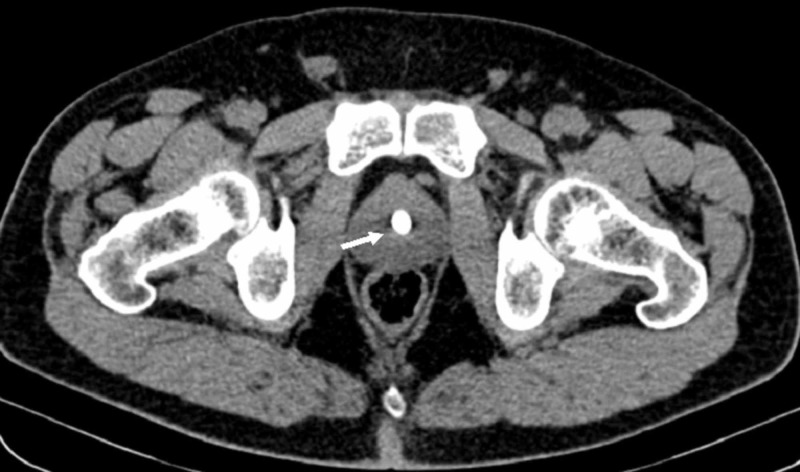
Unenhanced CT scan of the pelvis (axial view) showing the presence of a 9-mm calculi within the prostatic urethra CT, computed tomography
